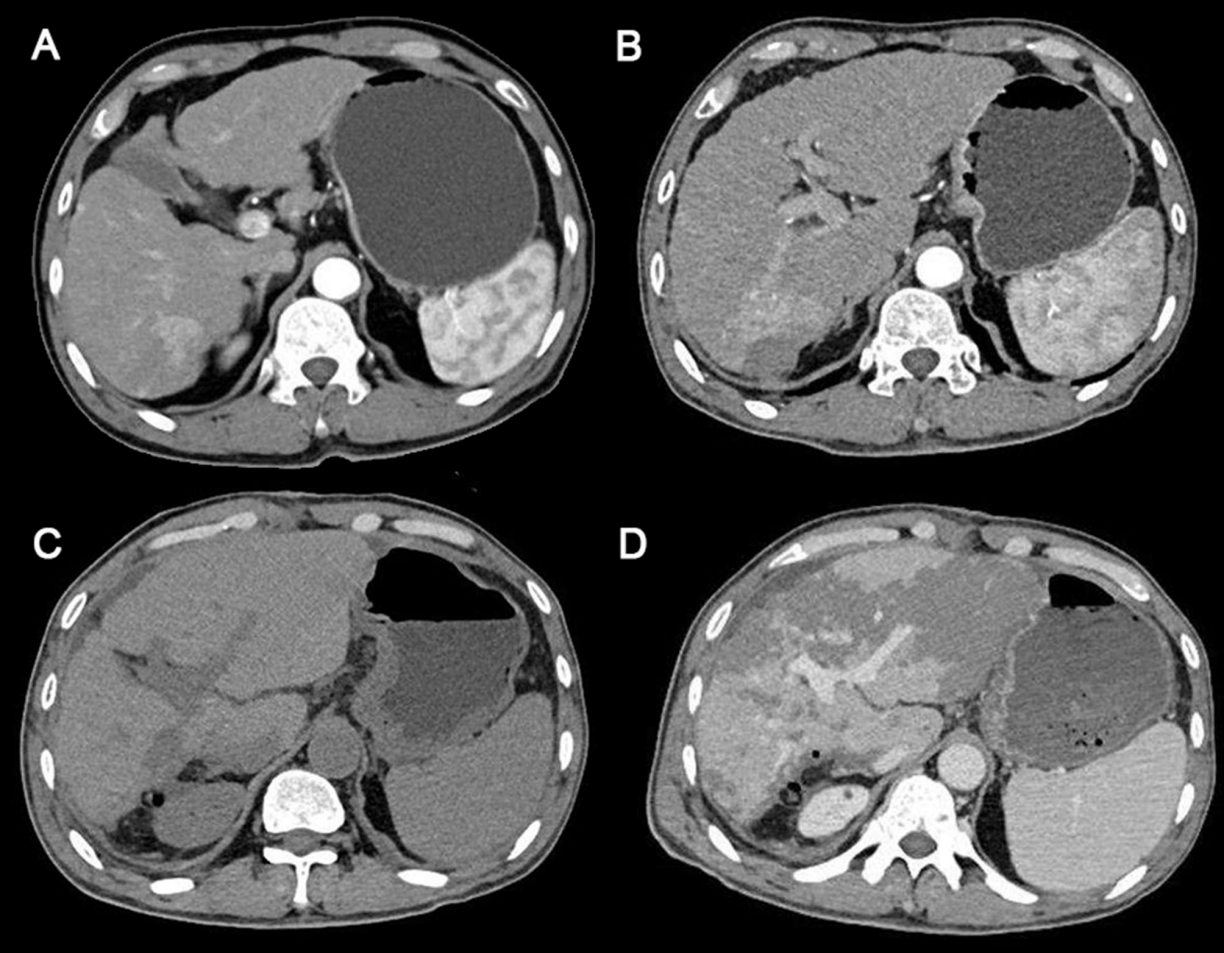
Abdominal computed tomography scans of the patient. (A) The time the patient was first diagnosed with HCC 2 years ago. (B) Before surgery. (C) The sixth day after surgery. (D) The eighth day after surgery (the day of PHLF onset). HCC, hepatocellular carcinoma; PHLF, posthepatectomy liver failure.
